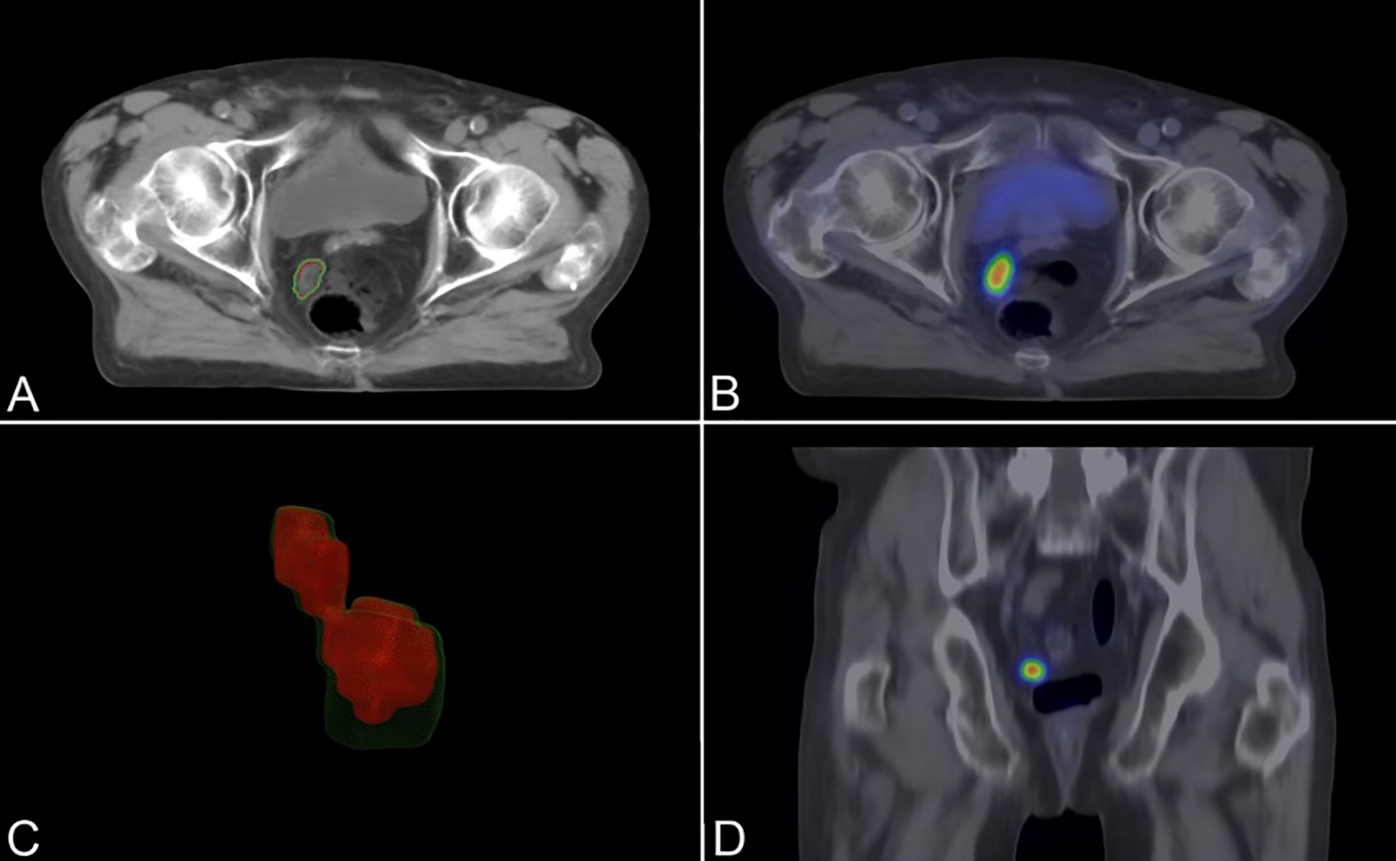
An 81-year-old patient with PSMA-positive enlarged pelvic lymph node (PSA 6.20 ng/ml; Gleason: 3 + 4 = 7). Topometric CT (axial slice (A)) fused and registered with ^18^F-PSMA-PET images (axial (B) and coronal slices (D)). Smoothed 3D polygon model of the GTV_CT_ (green outlines) and BTV_PSMA_ (red outlines) (C). PSMA, prostate-specific membrane antigen; PSA, prostate-specific antigen; GTV_CT_, gross tumor volume on the topometric CT; BTV_PSMA_, biological target volume on the PSMA-based examination.
